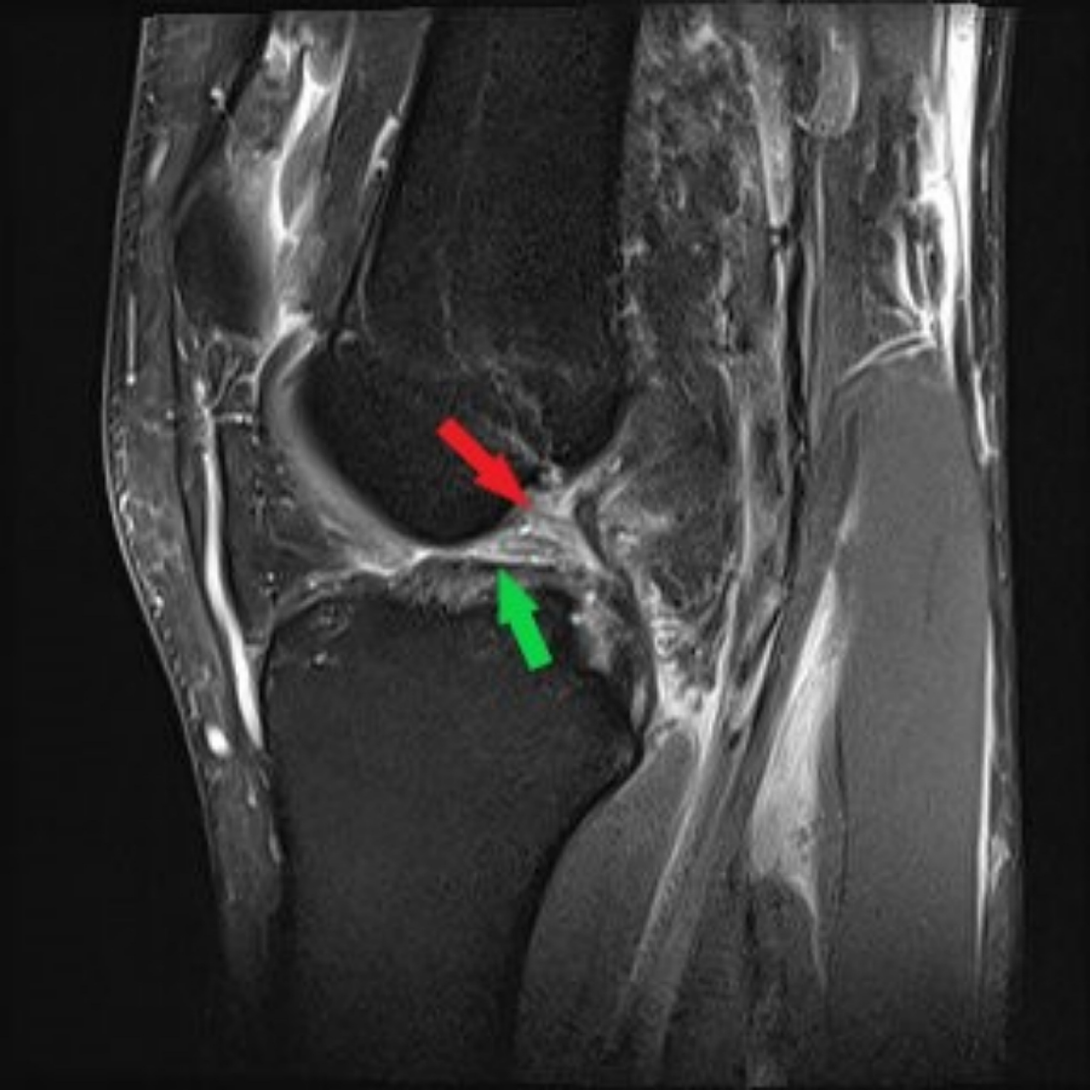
Sagittal T2-weighted MRI of the left knee in patient 1 Sagittal T2-weighted MRI of the left knee demonstrating complete disruption of the ACL (green arrow) and proximal detachment of the PCL (red arrow).
